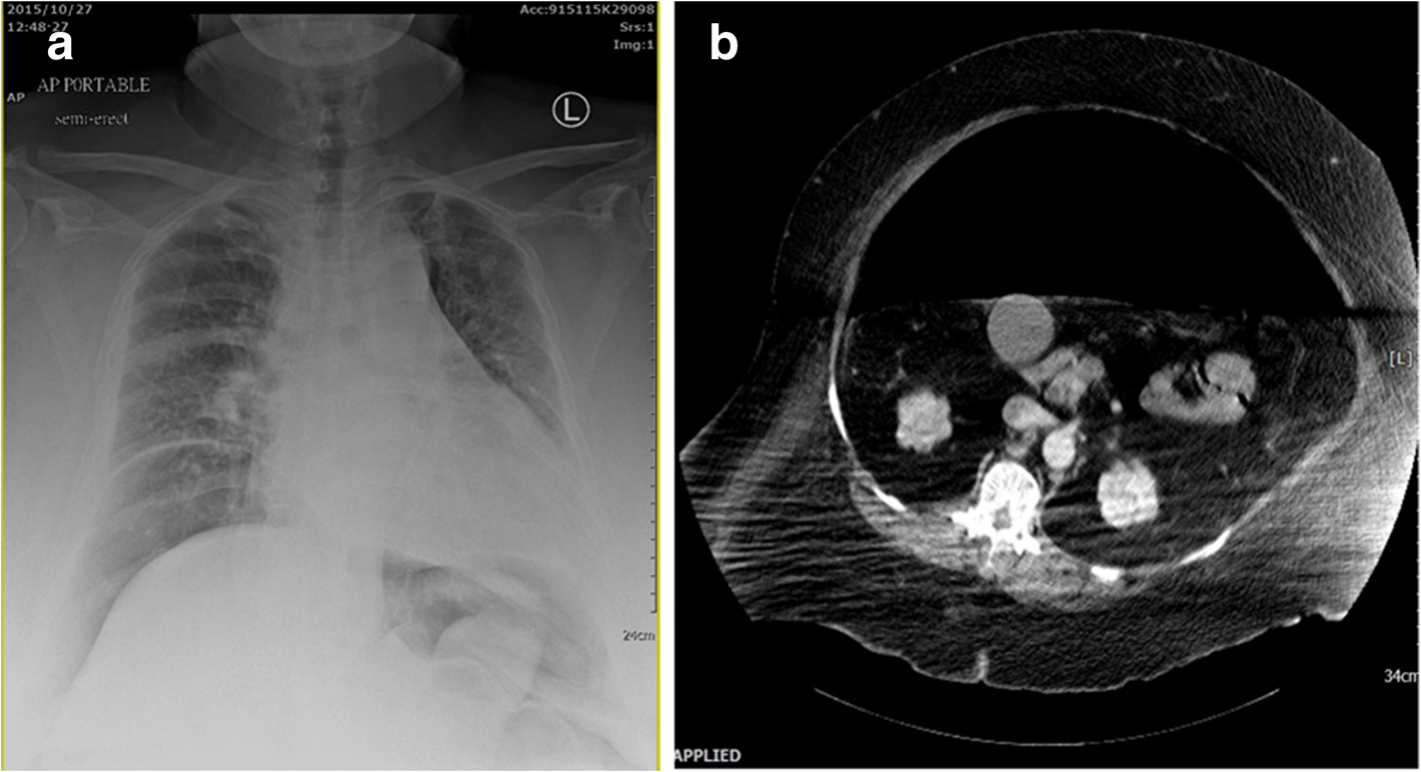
a Chest X-ray demonstrating an elevated diaphragm and suspicious pneumoperitoneum. b Computed tomography scan, transverse view. Massive free air and minimal ascites were noted in the abdomen
